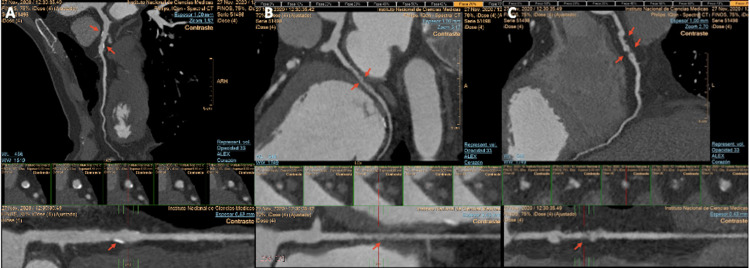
Coronary CT angiography findings of the patient A: left descending coronary artery; B: circumflex coronary artery; and C: right coronary artery with a diffuse multi-vessel disease with calcified and non-calcified plaque burden CT: computed tomography
